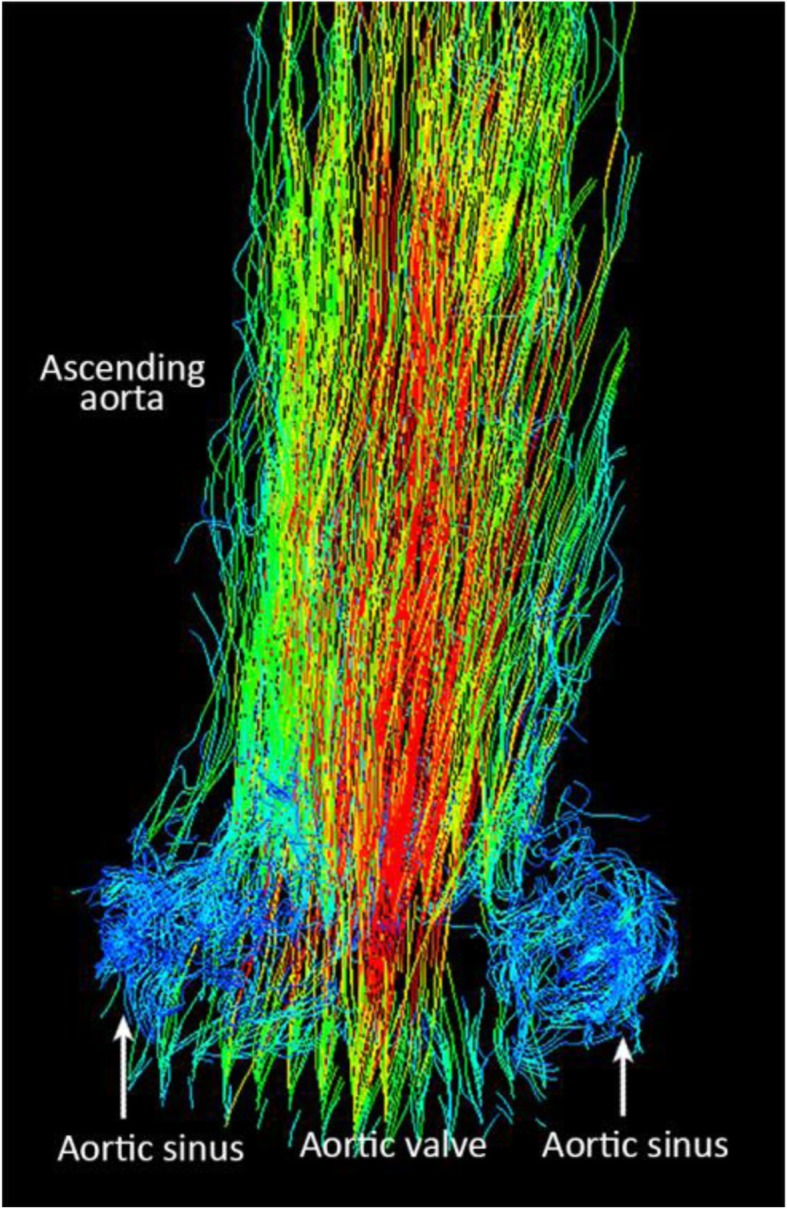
Particle tracing of flow from 4D phase-contrast MRI showing vortices in the aortic sinuses. Seeds were placed at the aortic valve orifice (Corresponding video file attached – Additional file 3: Video S3)
